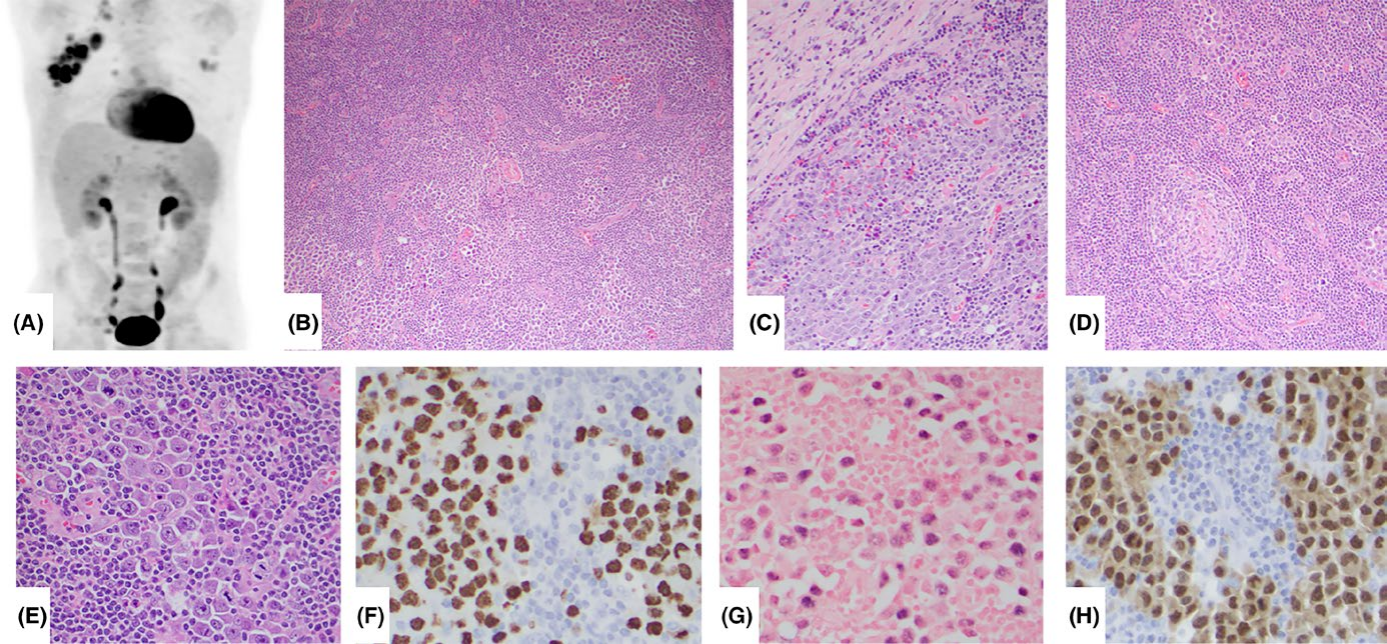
15‐fluorodeoxyglucose (FDG) positron emission tomography scan demonstrated multiple enlarged FDG‐avid regional lymph nodes (A). Lymph node excisional biopsy revealed multiple foci of neoplastic cells replacing some germinal centers (B). These cells were also present in mantle zones surrounding benign germinal centers, and other clusters were noted within sinusoids (C). Most residual lymphoid follicles were atretic and had hyaline‐vascular changes, “onion skin” mantle zones, and prominent interfollicular high endothelial venules (D). The neoplastic cells had plasmablastic morphology, characterized by large cells with vesicular eccentric nuclei, prominent nucleoli and abundant amphophilic cytoplasm (E). The neoplastic cells were positive for human herpesvirus 8 (HHV‐8) (F), Epstein‐Barr virus‐encoded RNA (G), and MUM1/IRF4 (H)
